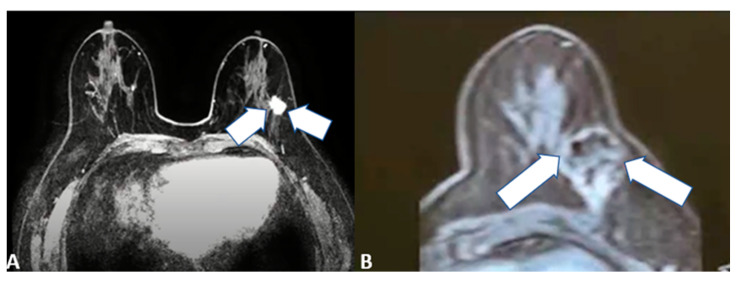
64-year-old female patient with left invasive T1 breast cancer of 11 mm with a molecular subtype Luminal-A. Contrast-enhanced T1-weighted MRI scan showing (A) baseline neoplastic lesion and (B) 5-month follow-up after microwave ablation with good ablation margins and central necrosis.
